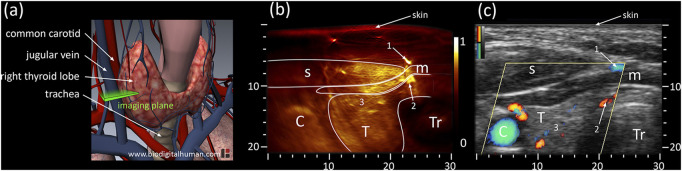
(A) Anatomy of thyroid including cardio-vascular and respiratory system; the cross-sectional imaging plane is highlighted in green. (B) PAT and (C) US cross-sections of the left thyroid lobe of the first volunteer. PAT: Photoacoustic tomography, US: Ultrasound; C: Carotid T: Thyroid, Tr: Trachea, s: sternocleidomastoid muscle, m: infrahyoid muscle; axes in mm. Reprinted with permission from [Dima A., Ntziachristos V. (2016). *In-vivo* handheld optoacoustic tomography of the human thyroid[J]. Photoacoustics, 4(2):65–69. doi: 10.1016/j.pacs.2016.05.003].
